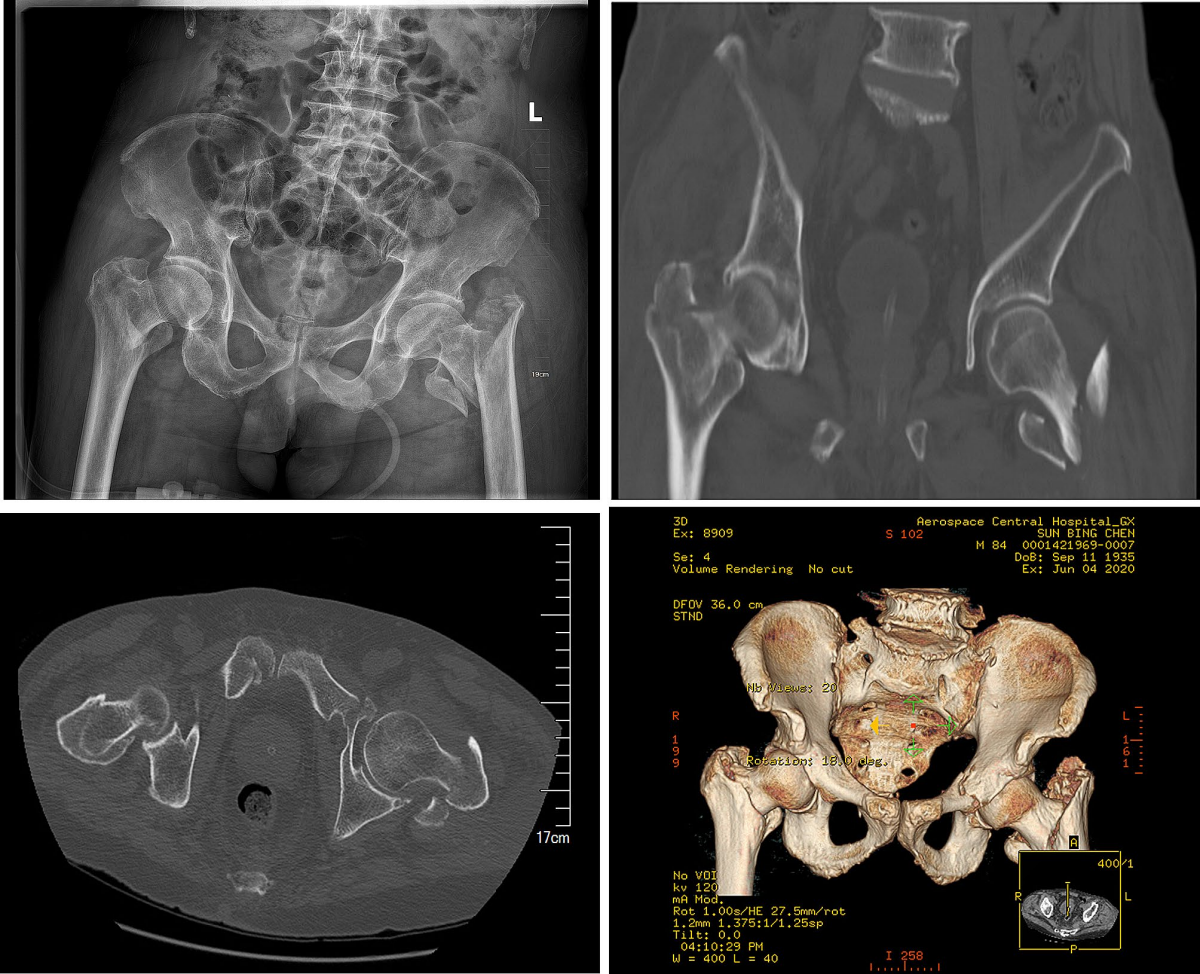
Preoperative imaging of the hip. X-ray and 3D showed bilateral intertrochanteric fractures of the femur, with the left fracture involving the greater and lesser trochanters, which are more obviously displaced, and the right shortened and displaced
